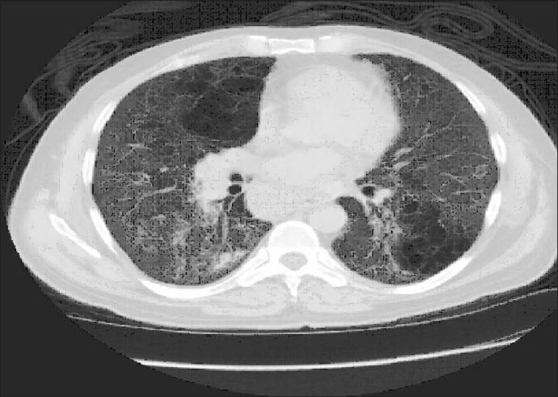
Patient 5. HRCT scan at the level of the left atrium demonstrating diffuse ground glass attenuation with fine reticulation and cystic changes. Lymph node enlargement involving the azygoesophageal recess is seen. Clinical characteristics of the patient are shown in Table 4
